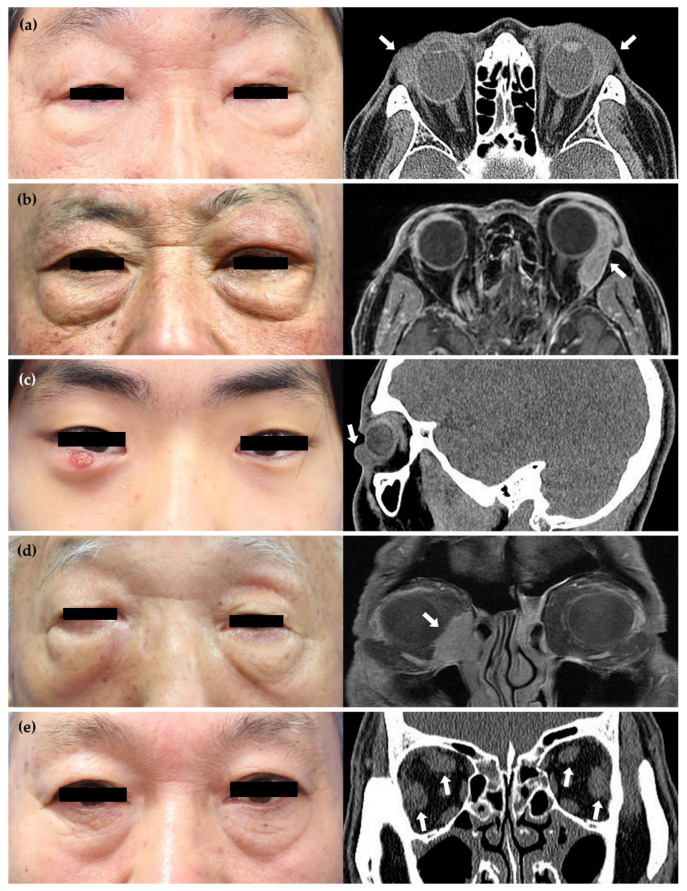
Gross photo (left) and radiological image (right) according to the 5 affected sites (white arrow) of IgG4-ROD. (a) Lacrimal gland: a 71-year-old male presented with bilateral eyelid swelling, and a facial CT showed enlargements of the bilateral lacrimal glands, more pronounced on the left. (b) Orbit: a 73-year-old male presented with swelling of the left eyelid, and an orbit MRI with CM showed a 3.3 × 1.2 × 1.9 cm lobulated enhancing mass in the superolateral aspect of the extraconal space in the left orbit. (c) Eyelid: a 20-year-old male presented with a mass in the right eyelid, and a facial CT revealed about 0.5 × 0.8 × 1.1 cm of soft tissue-attenuated swelling in the right lower eyelid. (d) Lacrimal sac: a 76-year-old male presented with swelling of the right eyelid, and an orbit MRI with CM showed a 3.0 × 2.5 cm lobulated soft tissue mass in the right anteroinferomedial orbit. (e) EOM: a 60-year-old male complained of diplopia, and a facial CT showed severe diffuse enlargements of the bilateral superior and lateral rectus muscles. IgG4-ROD, IgG4-related ophthalmic disease; CT, computed tomography; MRI; magnetic resonance imaging; CM, contrast media; EOM, extraocular muscle.
